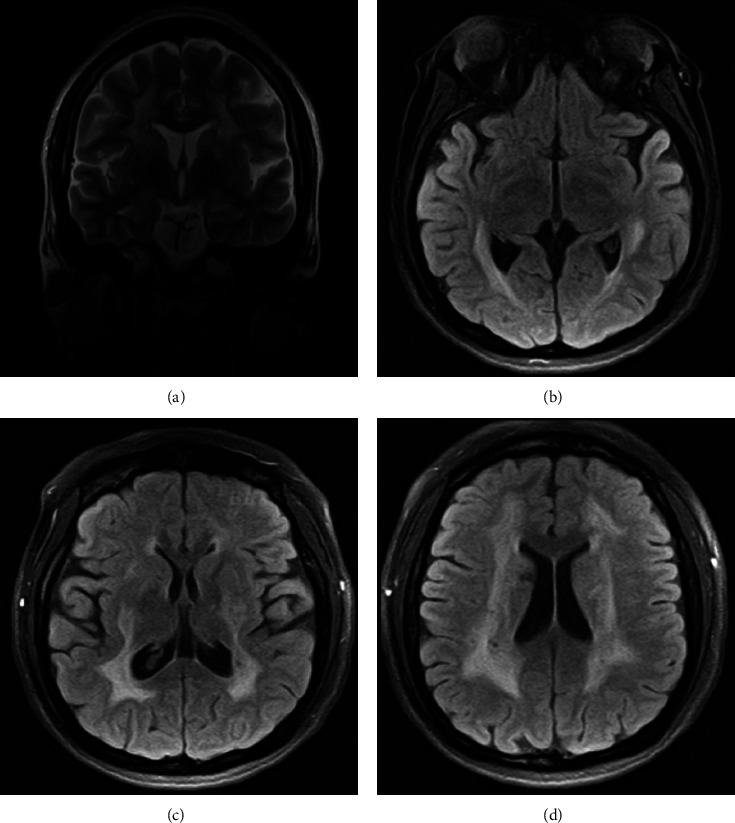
Brain 3.0 Tesla MRI. (a) Coronal section in the T2 sequence. (b–d) Axial sections in T2 FLAIR sequence showing a bilateral and symmetric posterior leukodystrophy, acute right frontal and parietal lacunar ischemic strokes, and chronic lacunar infarction in the left body of the caudate nucleus.
